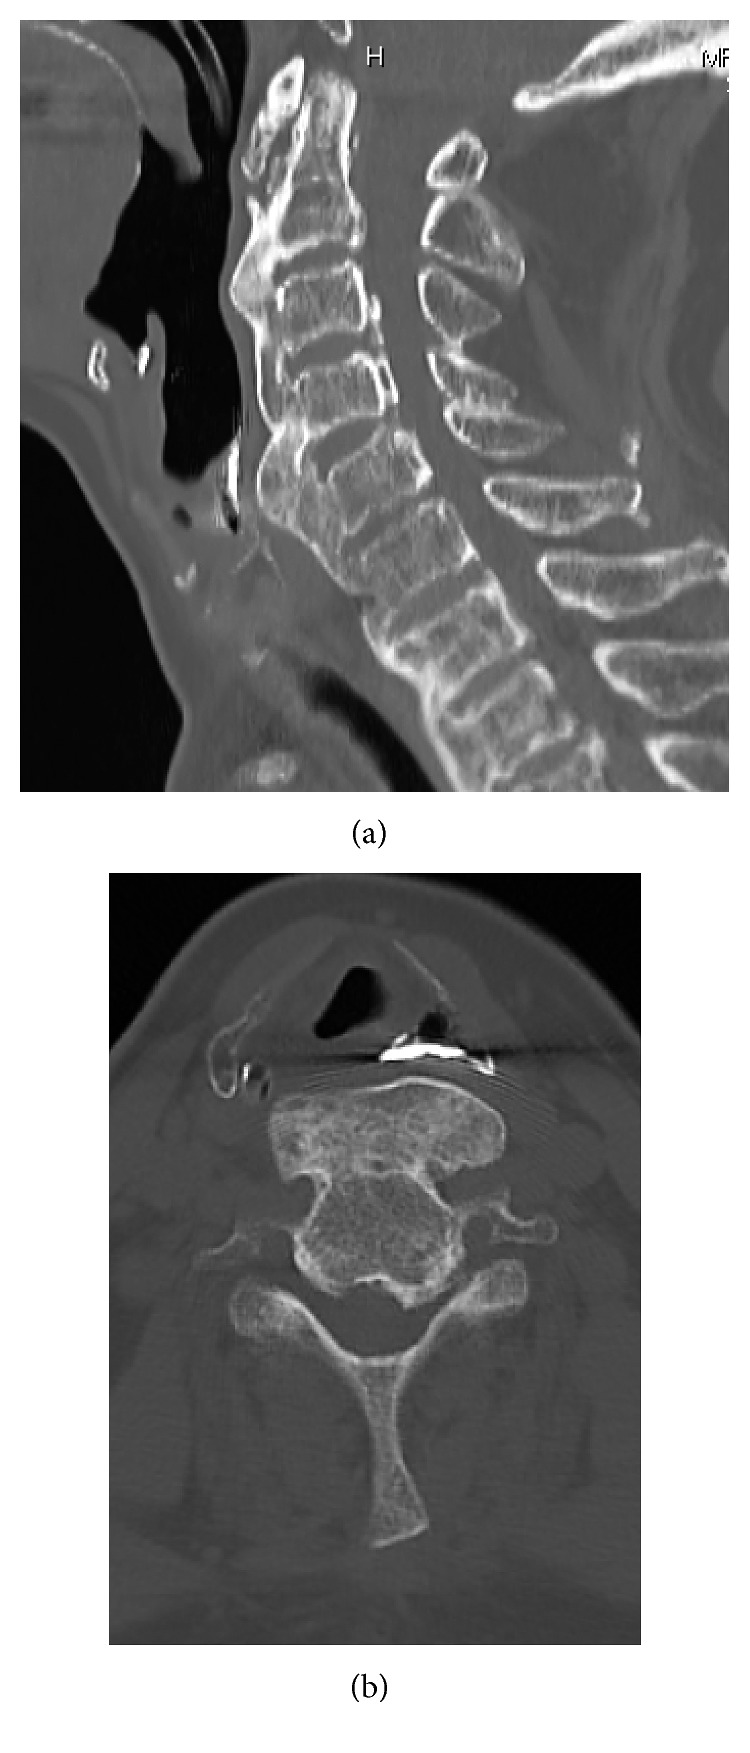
Sagittal (a) and axial (b) image of CT showing extensive OALL at C4-5.
